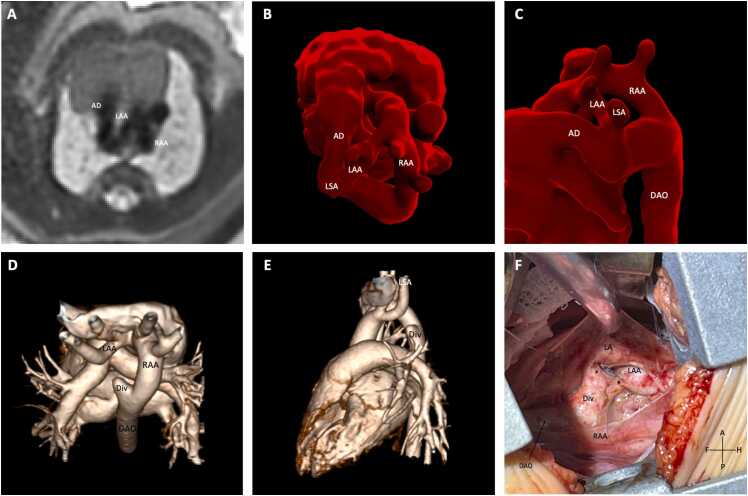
DAA with LAA isthmal atresia. Fetal CMR at 34 weeks (A) demonstrates the Z-sign on a transverse plane of the upper mediastinum, formed by a dominant RAA, smaller LAA, and left-sided AD which is a similar view to that seen on fetal echocardiography. The 3D mesh shows a tortuous left-sided AD and apparent complete DAA but with significant anterior displacement of LAA isthmus as demonstrated in superior (B) and left lateral views (C). Postnatal CT (D and E) at 4 months shows an incomplete DAA with LAA isthmal atresia distal to the left SA. Posterior course of the left arch, including tethering of left SA (E), four-vessel sign (D), and diverticulum arising from descending aorta (D), can be identified. Surgery at 9 months (F) demonstrated a (ligamentous) connection (asterisk) between left SA and descending aorta. *AD* arterial duct, *DAO* descending aorta, *Div* diverticulum, *LA* ligamentum arteriosum, *LAA* left aortic arch, *LSA* left subclavian artery, *RAA* right aortic arch, *DAA* double aortic arch, *CMR* cardiovascular magnetic resonance, *CT* computed tomography, *3D* three-dimensional.
